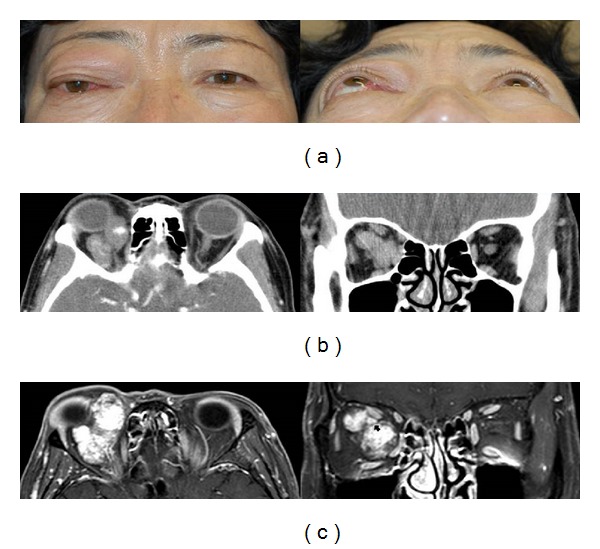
(a) Preoperative clinical photography shows the significant proptosis and inferolateral displacement of right eye. (b) CT scan of initial presentation shows well-demarcated enhancing intraconal vascular tumor in the right orbit. (c) 14 years later, MRI showing hypointense masses on T1-weighted sequence and hyperintense on T2-weighted sequence and demonstrating dense contrast enhancement. The optic nerve is surrounded and compressed by tumor (black arrow).
